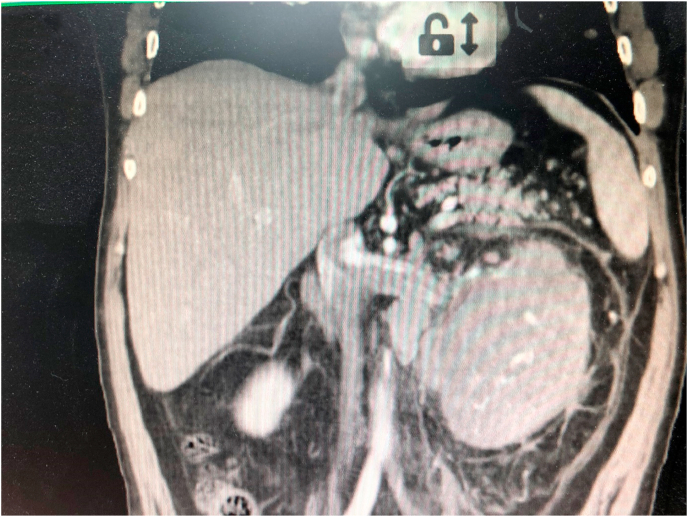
CT scan of left metastatic tumor of the kidney.
